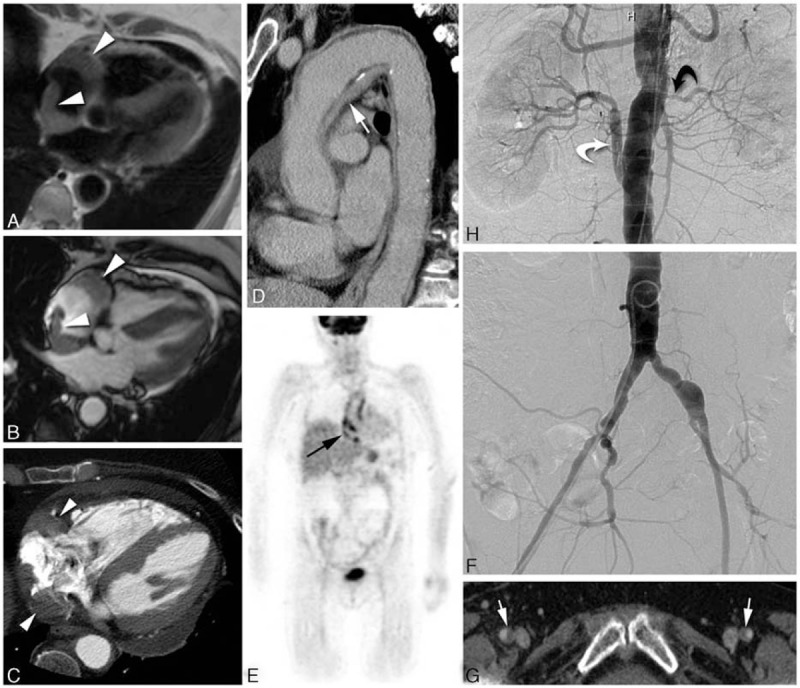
Diffuse cardiovascular involvement was observed. Cardiac MR (A, B) and contrast-enhanced cardiac CT (C) shows right atrial pseudotumor (white arrows). Contrast-enhanced thoracic CT (D) shows diffuse periaortic infiltration. ^18^FDG PET (E) shows abnormal periatrial and periaortic FDG uptake. DSA images (F) show diffuse aorto-iliac irregularity, left renal artery stent (black curved arrow), and right aorto-renal bypass (white curved arrow). Contrast-enhanced pelvic CT (G) shows bilateral femoral arteries infiltration. CT = computerized tomography, DSA = digital subtraction angiography, FDG = fluorodeoxyglucose, MR = magnetic resonance, PET = positron emission tomography.
